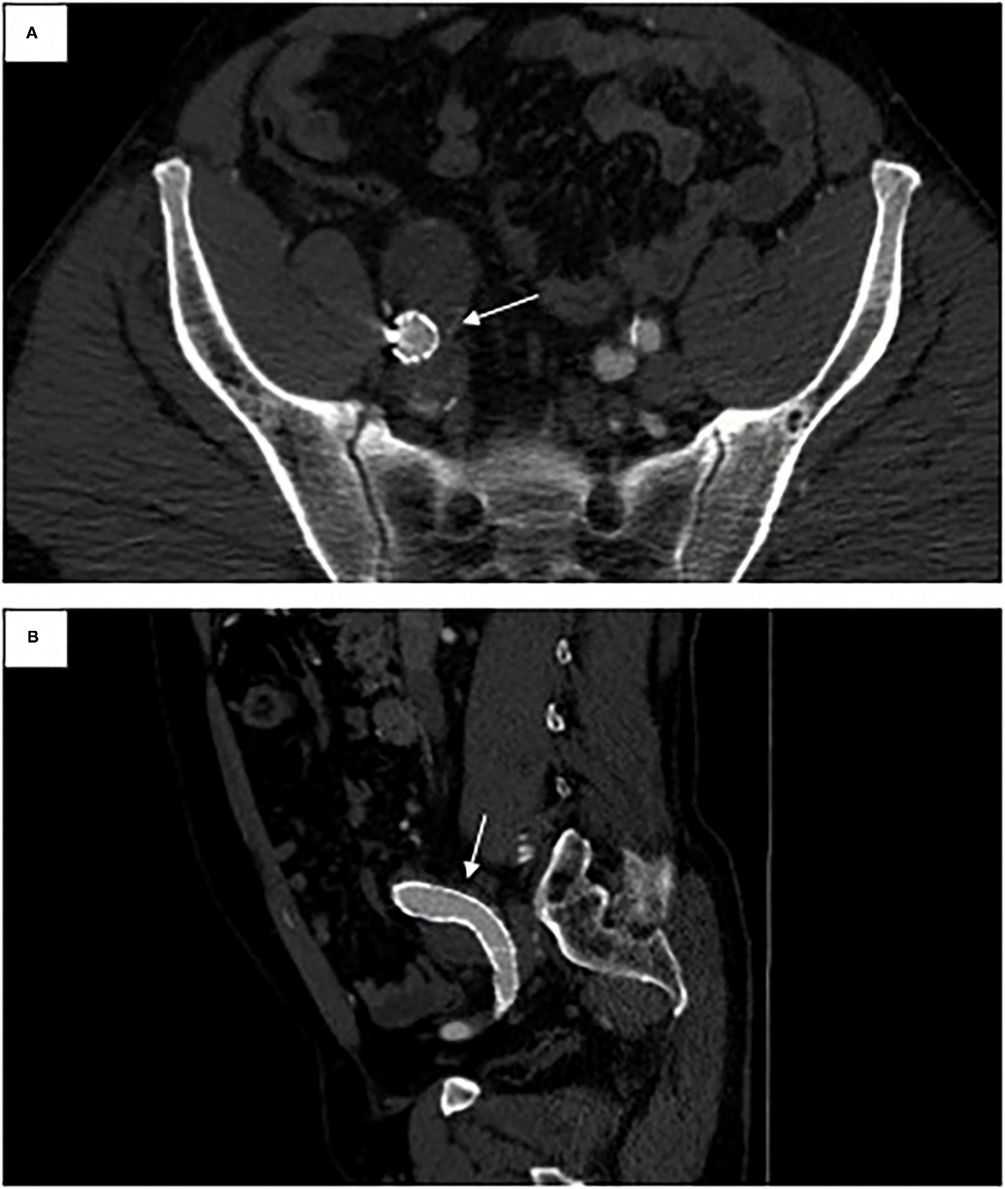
One-year follow-up CT angiogram. (A) Axial view of right iliac limb in previous fistula location. (B) Sagittal view of right iliac limb in previous fistula location.
